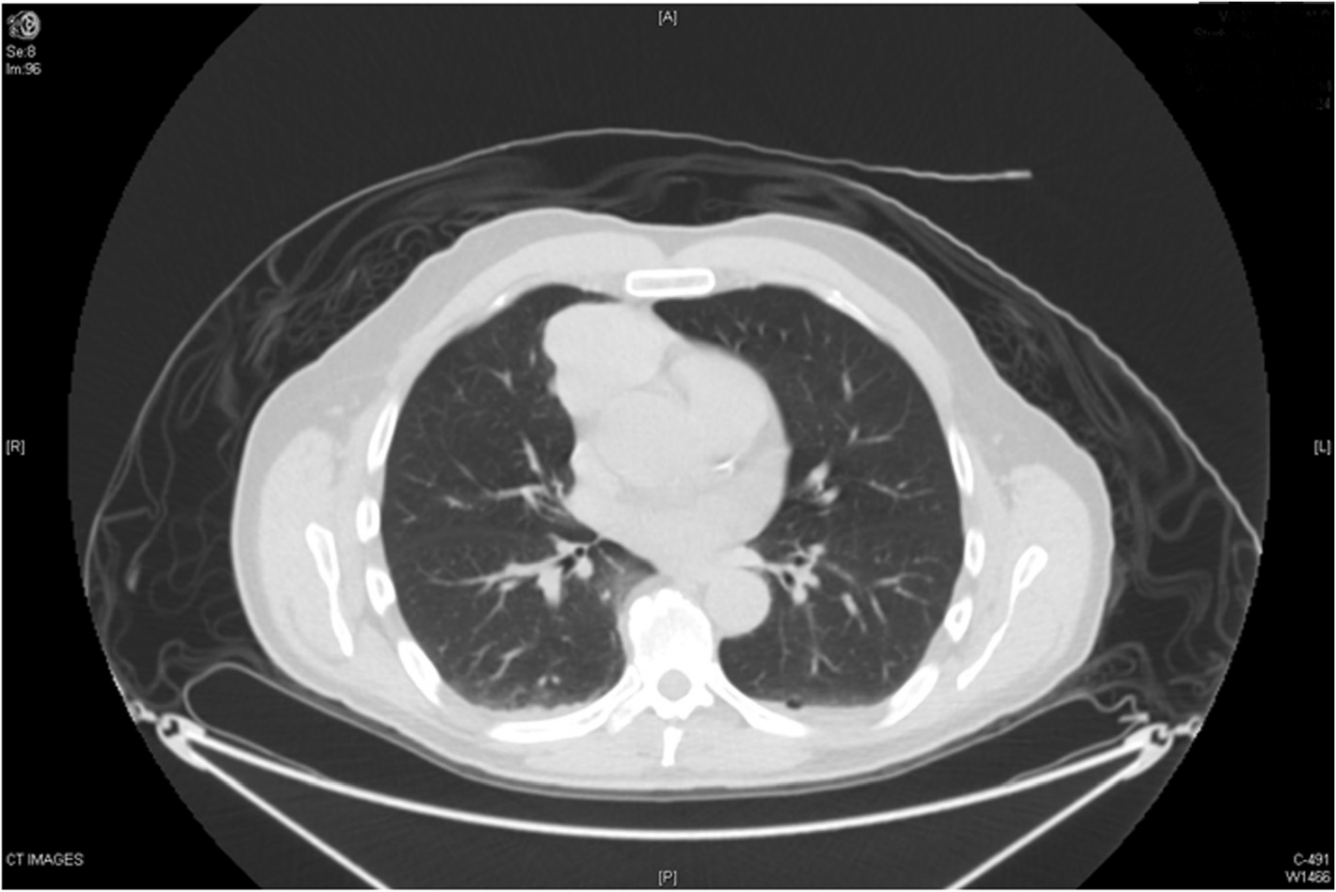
Axial section of initial CT scan with the anterior mediastinal mass abutting the pericardium.
